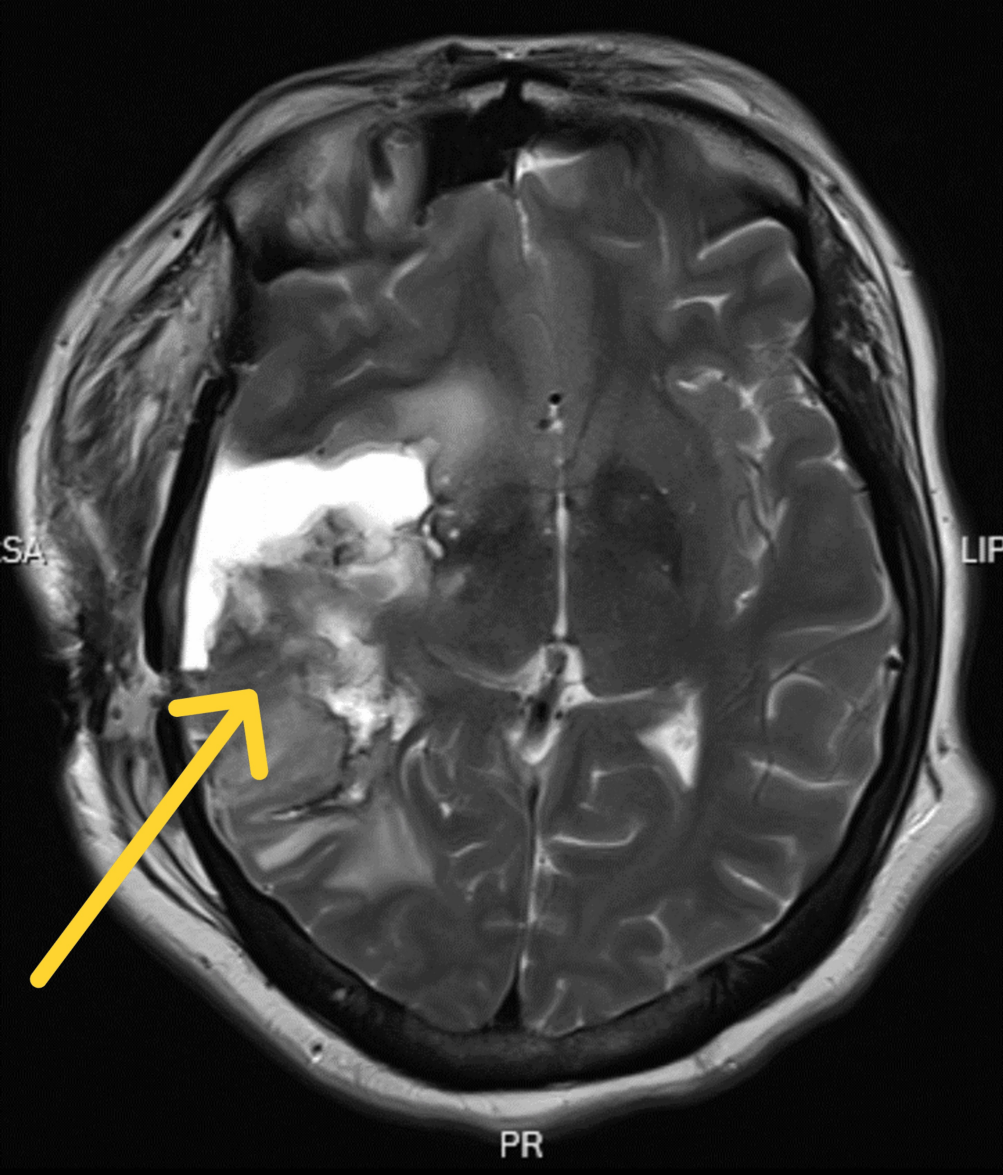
Postoperative MRI (T2 axial view) following redo craniotomy and tumor resection.
